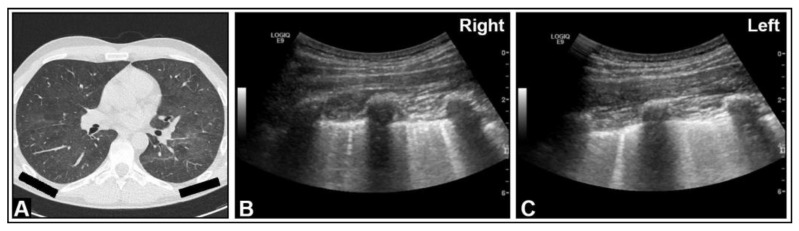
An HIV-positive patient with dyspnea and *Pneumocystis carinii* pneumonia. (A) On chest computed tomography, diffuse ground-glass opacities are observed. (B,C) On B-mode ultrasound, B-lines are visible in the right and left lungs.
